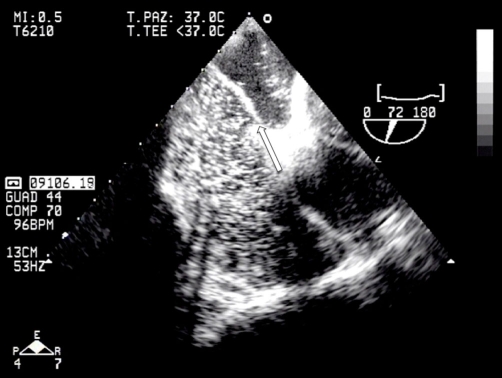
Transesophageal echocardiographic image of interatrial septum’s aneurysm with patent forame ovale.
